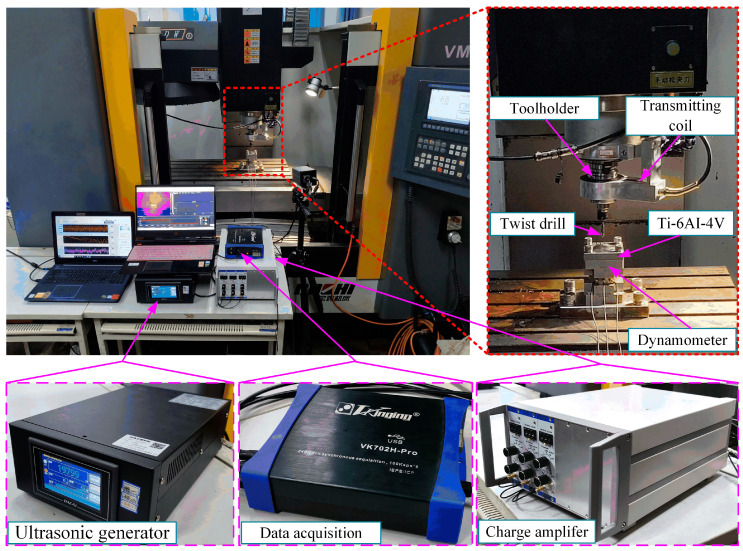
Ultrasonic vibration-assisted drilling experimental equipment.
